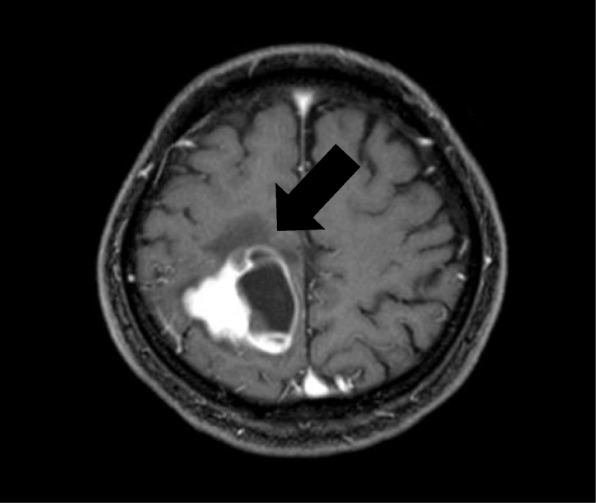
Brain magnetic resonance imaging (MRI) reveals a cerebral ring-enhancing lesion with hemorrhage (arrow)
